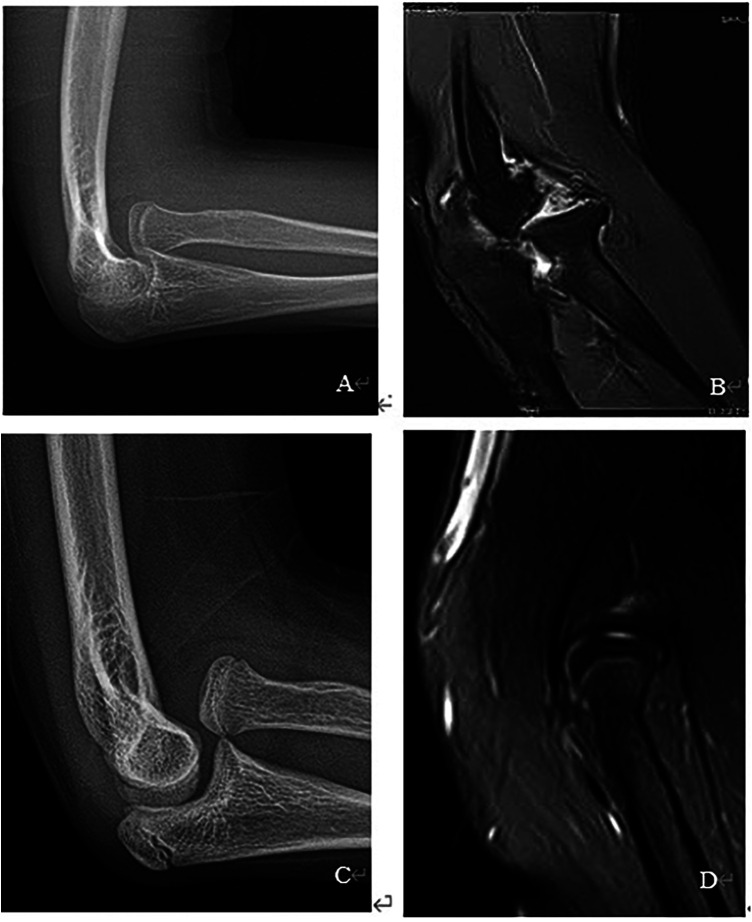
(A) Flat radial head in X, (B) flat radial head in MRI, (C) domed radial head in X, (D) domed radial head in MRI.
